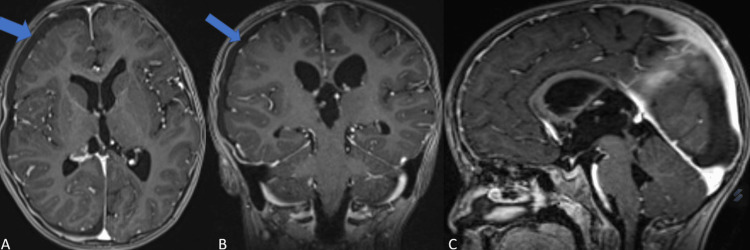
Postoperative contrast-enhanced MRI of a supratentorial ependymoma. A: Axial scan showing the complete removal of the tumor. All the ventricles appear relieved. As a secondary effect of surgery, the right frontoparietal subarachnoid space is enlarged (blue arrows). The enlargement can be defined as postoperative subdural hygroma (blue arrows). B: Coronal scan showing the gross-total resection. No residual tumor sign was observed. The right frontoparietal subarachnoid space was enlarged (blue arrow). C: Sagittal scan showing a marked regression in accompanying triventricular hydrocephalus and total regression in transependymal periventricular edema.
